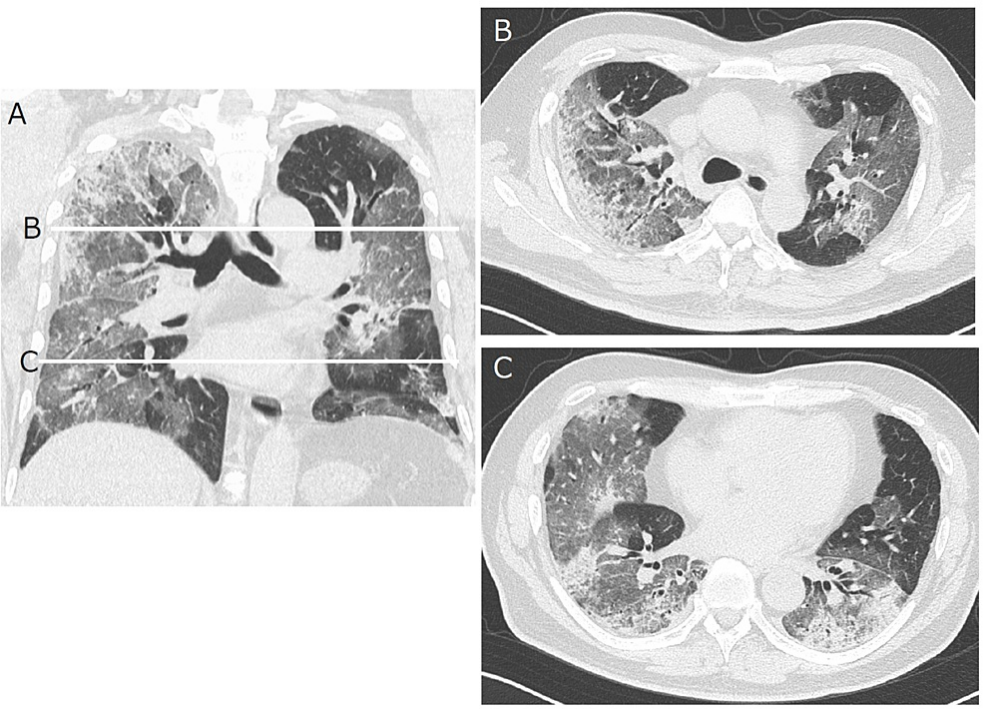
Chest CT imaging of typical COVID-19 pneumonia. Bilateral GGO dominant in the lower lobe and subpleural area makes a score of "3" using the Fukuchi-Manabe score (A) Coronal chest CT images (B). Just above the tracheal bifurcation level. (C) Heart level CT: computed tomography, COVID-19: coronavirus disease 2019, GGO: ground-glass opacity
